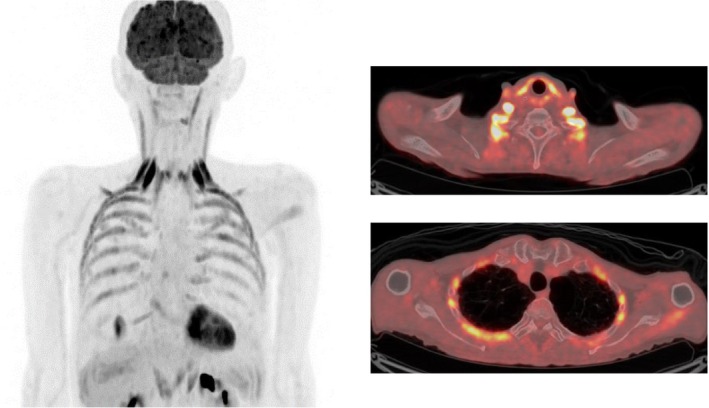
The 3D maximum intensity projection and fused positron emission tomography–computed tomography revealed significant ^18^F‐FDG uptake in the sternocleidomastoid and upper intercostal muscles and weak uptake in the diaphragm and lower intercostal muscles. Acquired at 120 kVp and 500 ms.
